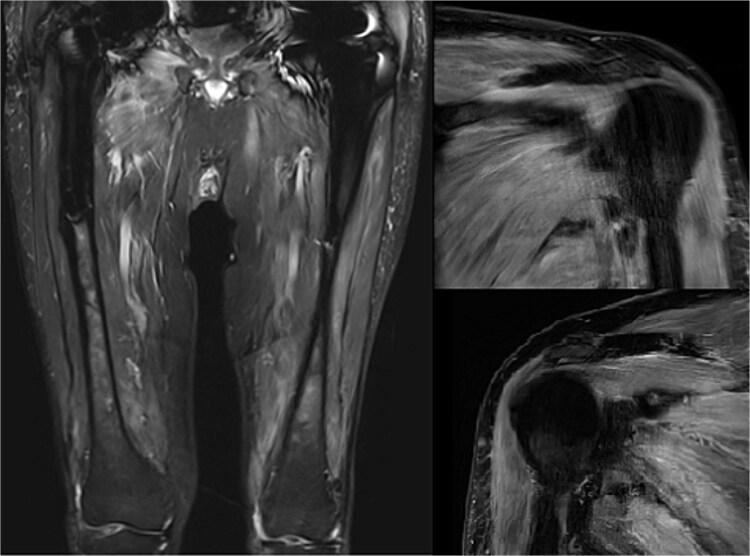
T2 STIR magnetic resonance imaging sequences of the shoulders and femurs demonstrating increased signal intensity. These findings are consistent with acute inflammatory myopathy as opposed to steroid associated myopathy, in which muscle atrophy and fatty infiltration with hyperintensity on T1-weighted sequences would be expected.
